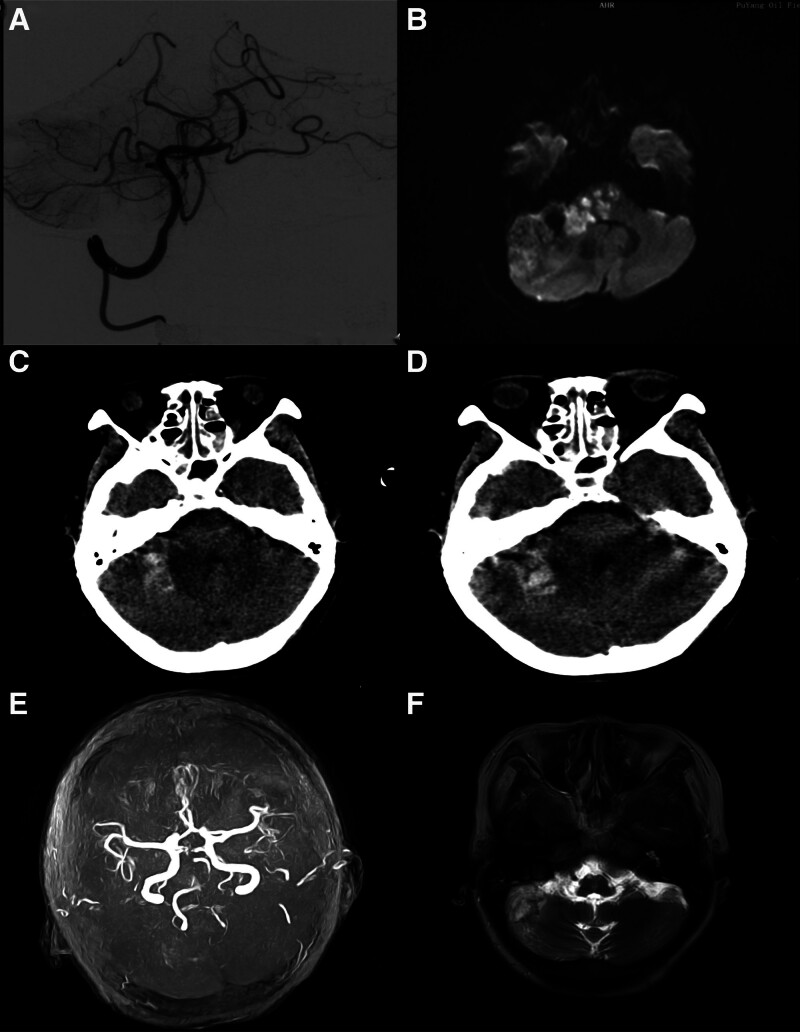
(A) Blood flow in the basilar artery was restored after mechanical thrombectomy (mTICI III). (B) MRI of the patient at 48 h after the operation showing punctate hemorrhage in the right cerebellar hemisphere, pons and pontine arm with multiple infarcts. (C and D) Cranial CT image indicating high-density signs in the right cerebellar hemisphere and pons, suggestive of localized hemorrhagic changes. (E) MRA image indicating patency of the basilar artery lumen at 4 months after surgery. (F) MRI examination revealed no new infarcts after 4 months of follow-up.
